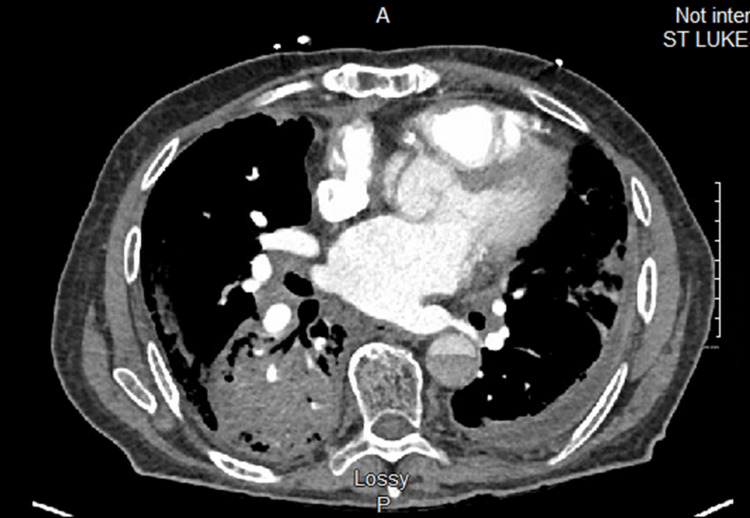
Initial patient CT angiography. Opacification of the pulmonary arteries without filling defect identified to suggest pulmonary emboli. There is a new dense consolidation in the right lower lobe. There is a slight increase in the amount of infiltrate in the right upper lobe posteriorly and the right middle lobe inferiorly. The prior left upper lobe infiltrate has improved. A stable amount of bilateral pleural effusion can be noted. Ascending aortic repair is stable. Arch and descending aortic dissection are again identified, continuing into the upper abdomen, stable in appearance. The visualized portions of the upper abdomen appear unchanged.
